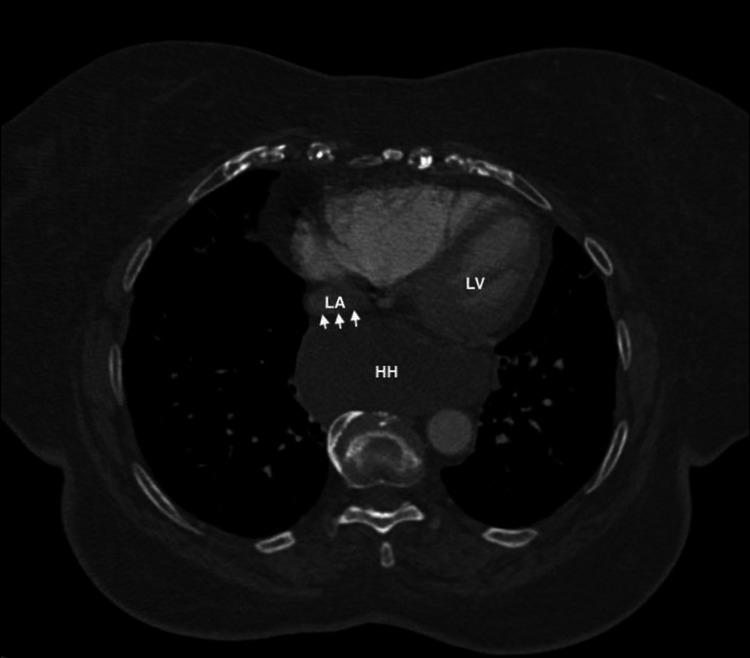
Axial view of CT chest showing compression of left atrium by a giant hiatal hernia. Arrows demonstrating the extrinsic compression of the left atrium by the giant hiatal hernia. LA: left atrium; HH: hiatal hernia; LV: left ventricle
